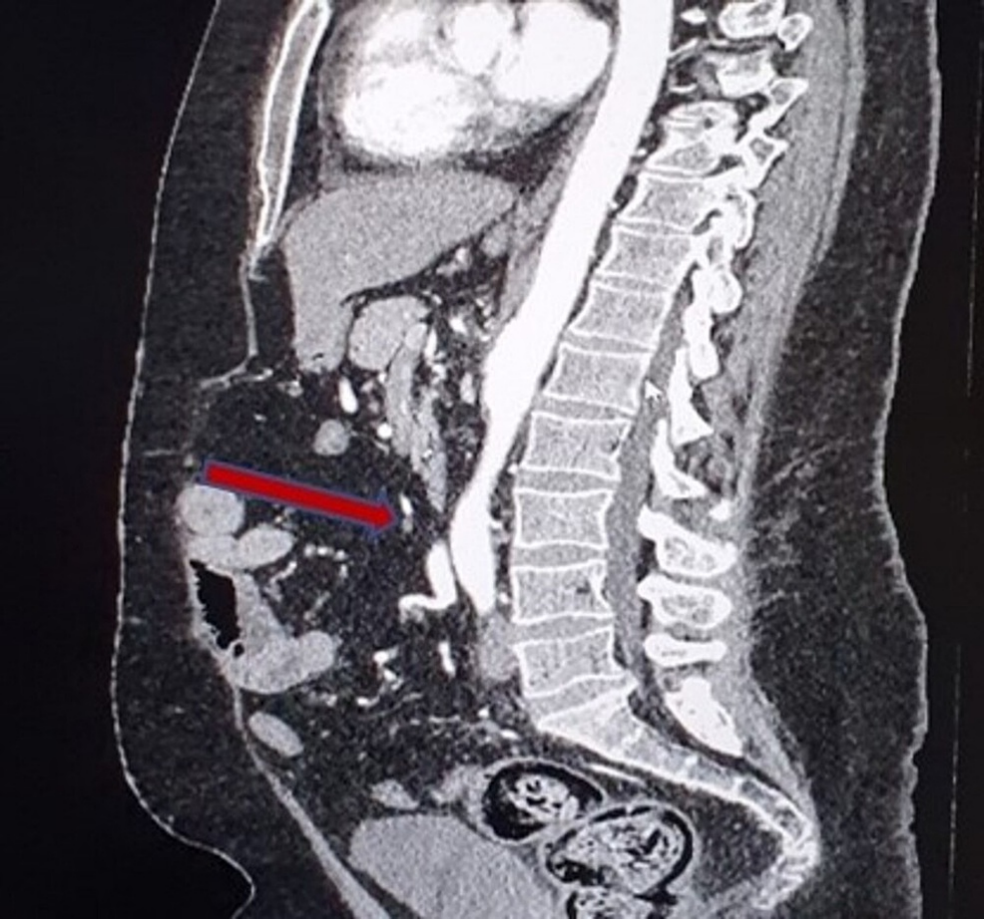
CT angiogram (sagittal view). Red arrow indicates narrowing at the origin of the common iliac artery.
